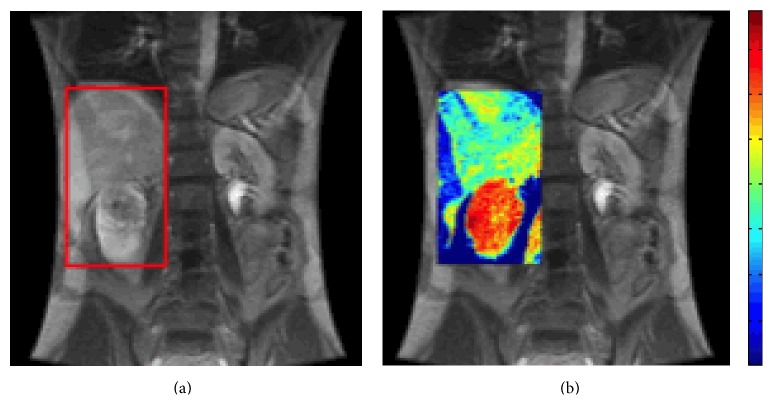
Perfusion MRI. T1-weighted MRI (a) and dynamic contrast-enhanced pMRI (b) of a renal mass: a series of 3D images were acquired. Each 3D image consists of 8 coronal slices with a 5-second acquisition time. Subsequently, 3D perfusion parametric map showing the microcirculation of the renal mass was generated. Red color in the tumor is indicative of a high level of perfusion. Surgical pathology revealed clear-cell RCC, Fuhrman grade 4, with sarcomatoid features.
